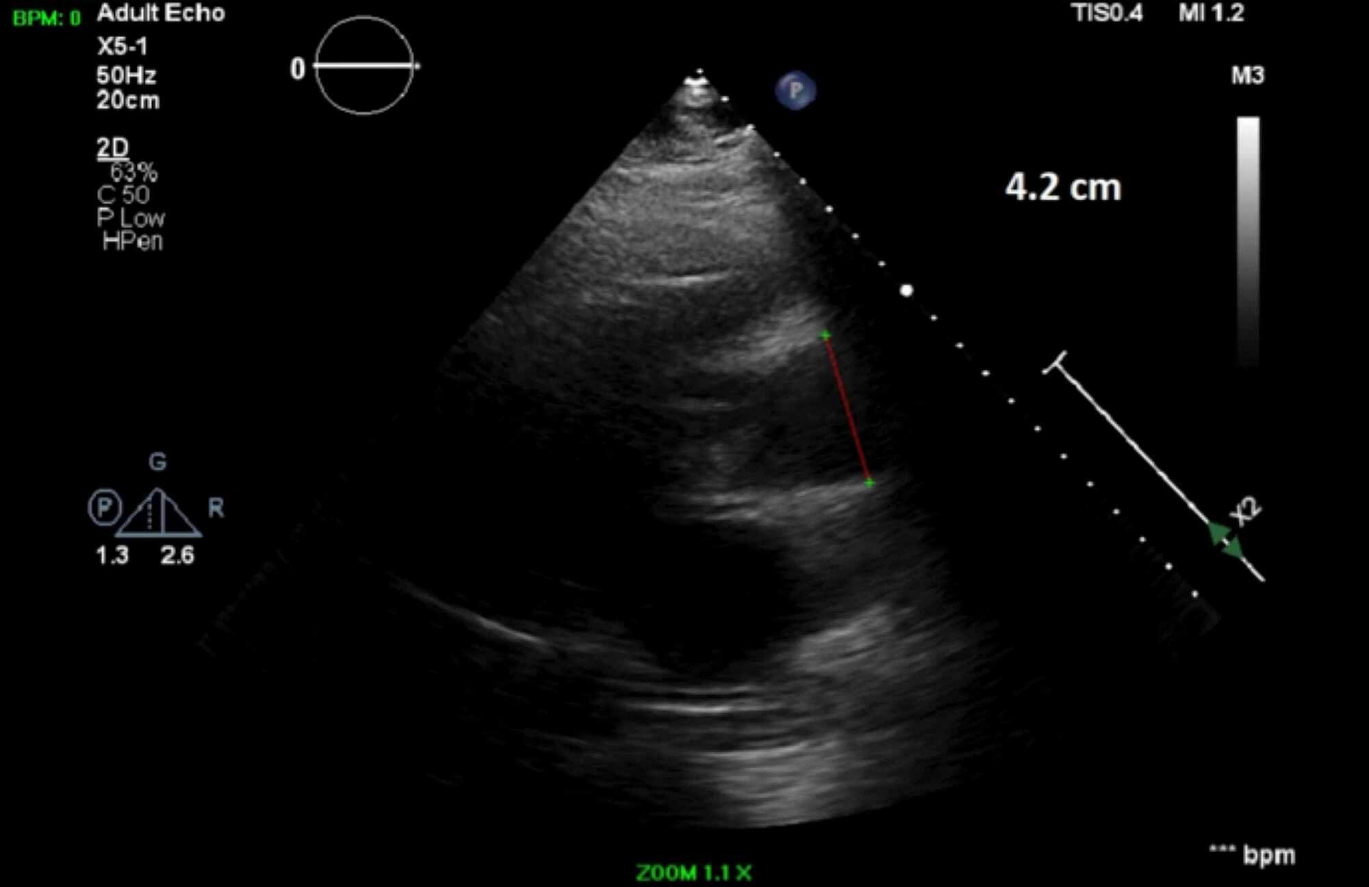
Transthoracic echocardiogram showing dilated ascending aorta of 4.2 cm
